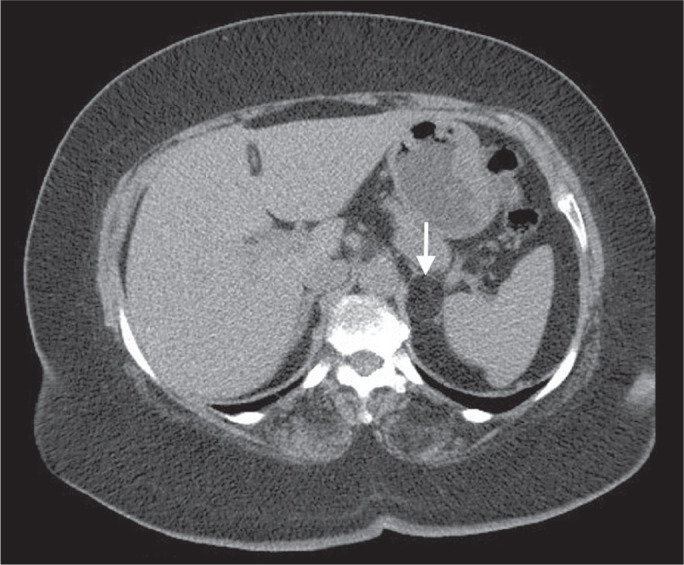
Adrenal myelolipoma in a 60-year-old woman. Unenhanced axial CT image
